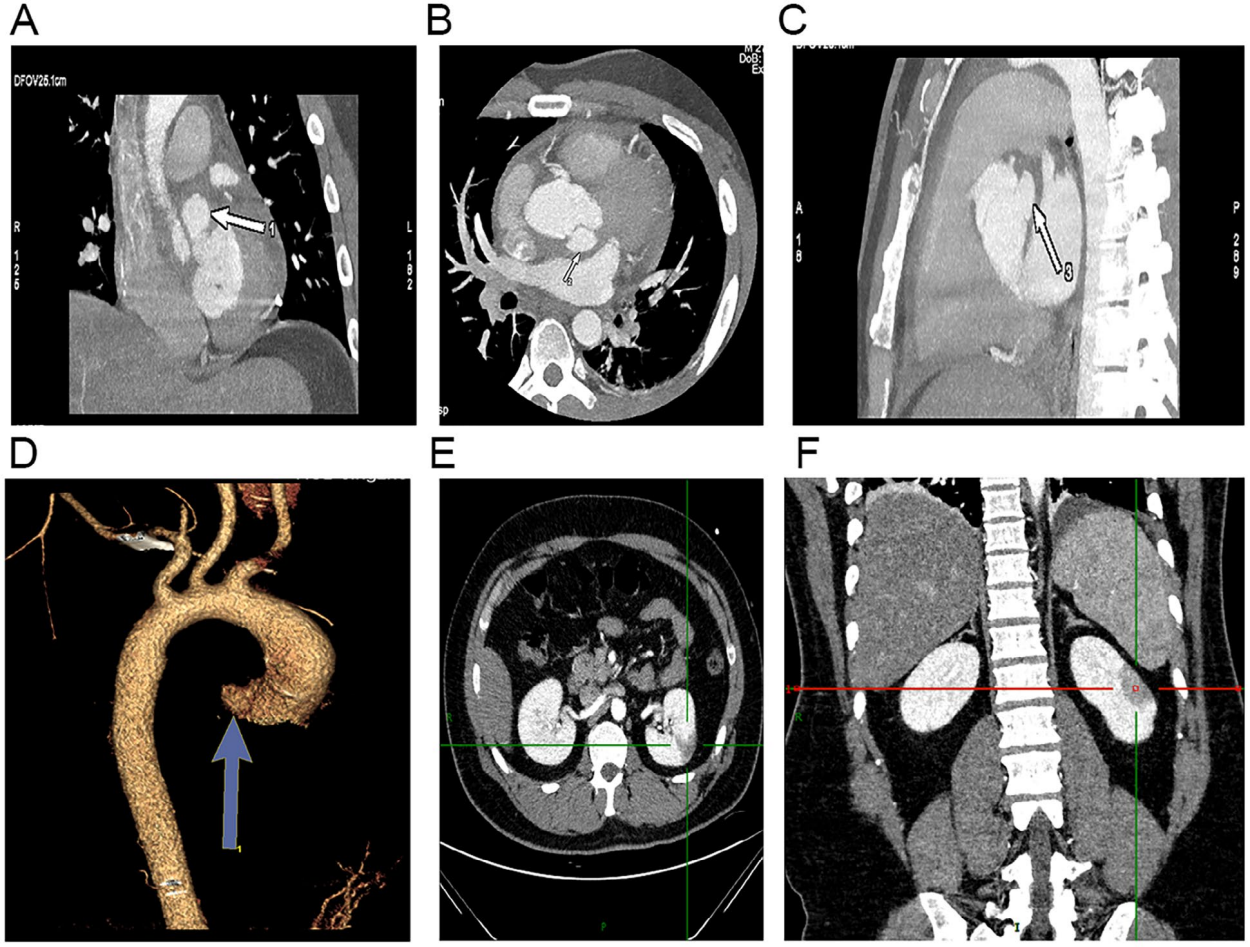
The images of the cardiac CT. (A) The coronal view of the heart, arrow points to the aneurysm of the aortic sinus. (B) The transverse section of the heart. (C) The sagittal view of the heart. (D) 3D image. (E) The transverse section of the kidney, the line indicates the area of renal infarction. (F) The coronal section of the kidney.
